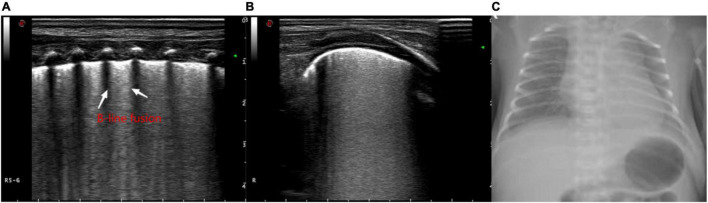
The ultrasonographic manifestations in the lungs of a newborn with alveolar-interstitial syndrome, showing the increase of B lines and the fusion into “white lung” like changes. (A) Longitudinal image of the intercostals. (B) Transverse image along the intercostals. (C) X-ray manifestation of the decrease of light transmission in both lungs.
